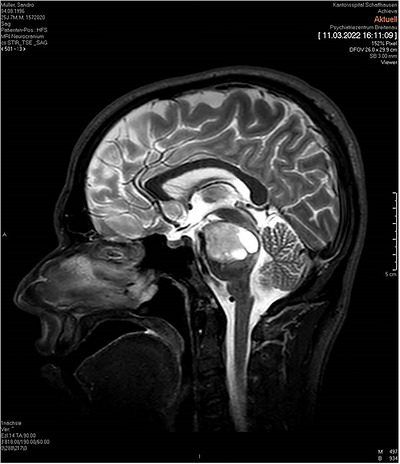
Brain MRI. T2w STIR sagittal: Partially cystic tumor in the significantly swollen pons (the “brightest” parts are the cysts).
